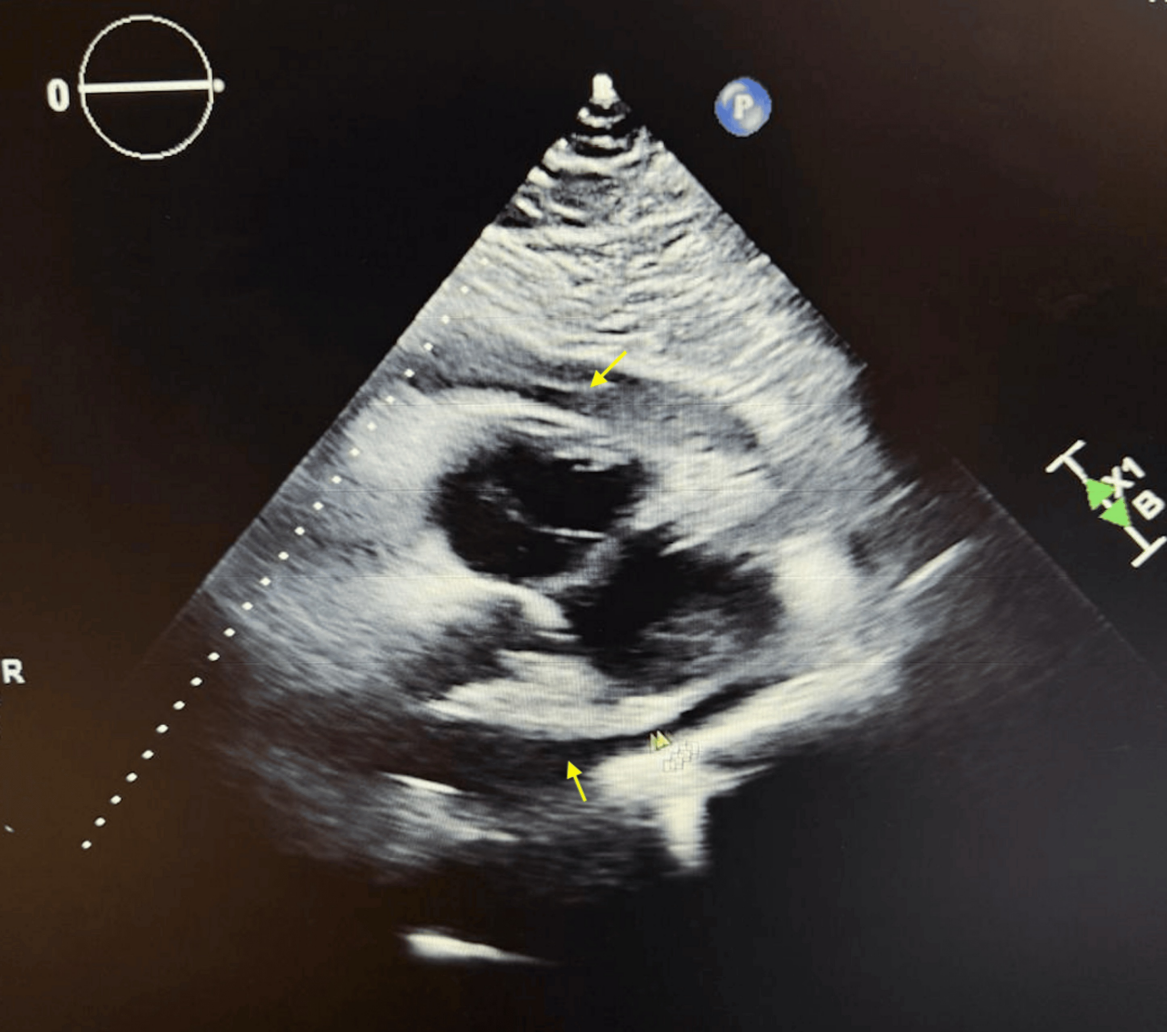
TTE (parasternal long-axis view): Circumferential pericardial effusion (yellow arrows) TTE: Transthoracic echocardiogram
